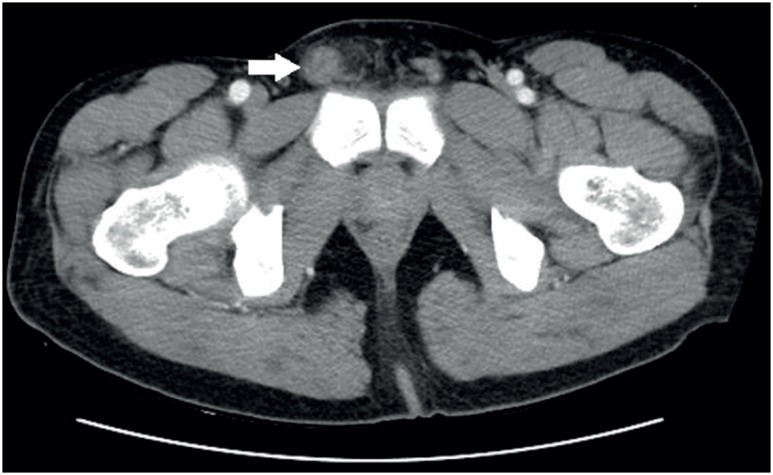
Axial computed tomography showing edematous changes and peripheral fat stranding on right spermatic cord.
